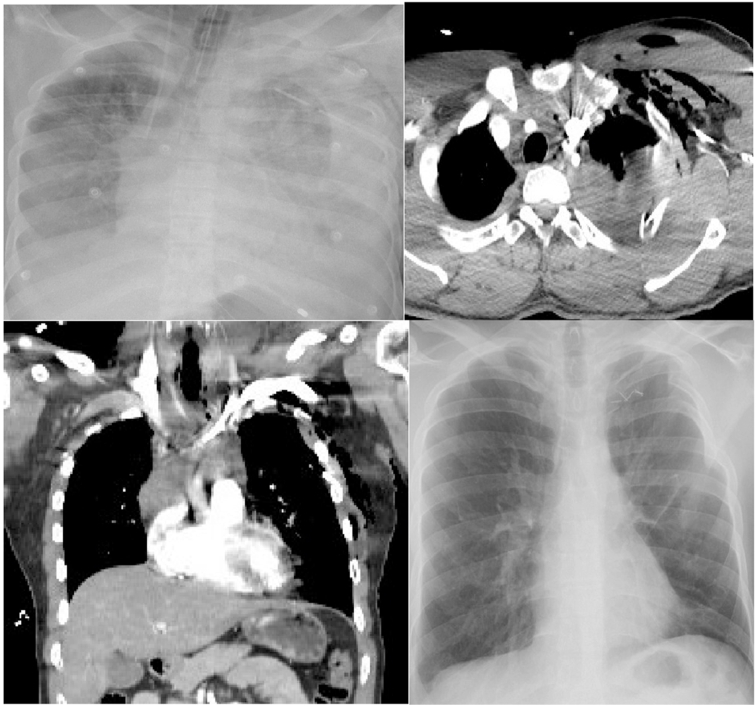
(A) Chest x-ray showing left hemothorax and chest drain. (B) CT scan of thorax axial view showing leakage of contrast in left upper chest most likely from subclavian artery injury and hemothorax (c) CT scan thorax coronal view showing some contrast leak (D) Post operative Chest -ray unremarkable showing some surgical clips.
